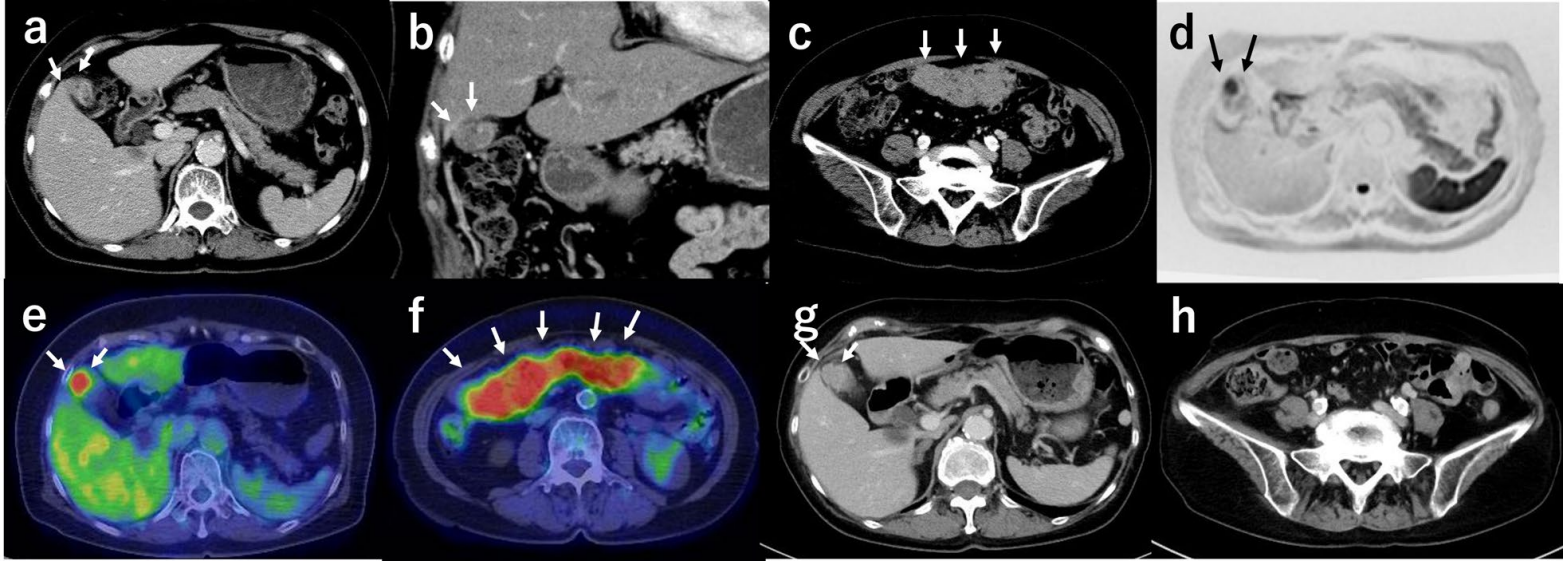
a, b, c Initial abdominal enhanced computed tomography (CT) in axial (a) and coronal (b) sections showed thickening with contrast enhancement of the gallbladder fundus (arrows) and multiple nodules in the peritoneal cavity (c). d Diffusion-weighted magnetic resonance imaging showed diffusion impairment at the primary tumor (arrows). e, f PET-CT showed high maximum standardized uptake values for the gallbladder tumor (e) and other nodules (f) (arrows). g, h After chemotherapy, abdominal CT showed that the primary tumor had enlarged slightly (g) (arrows), but that the peritoneal nodules had disappeared in some areas and had not increased in number (h)
